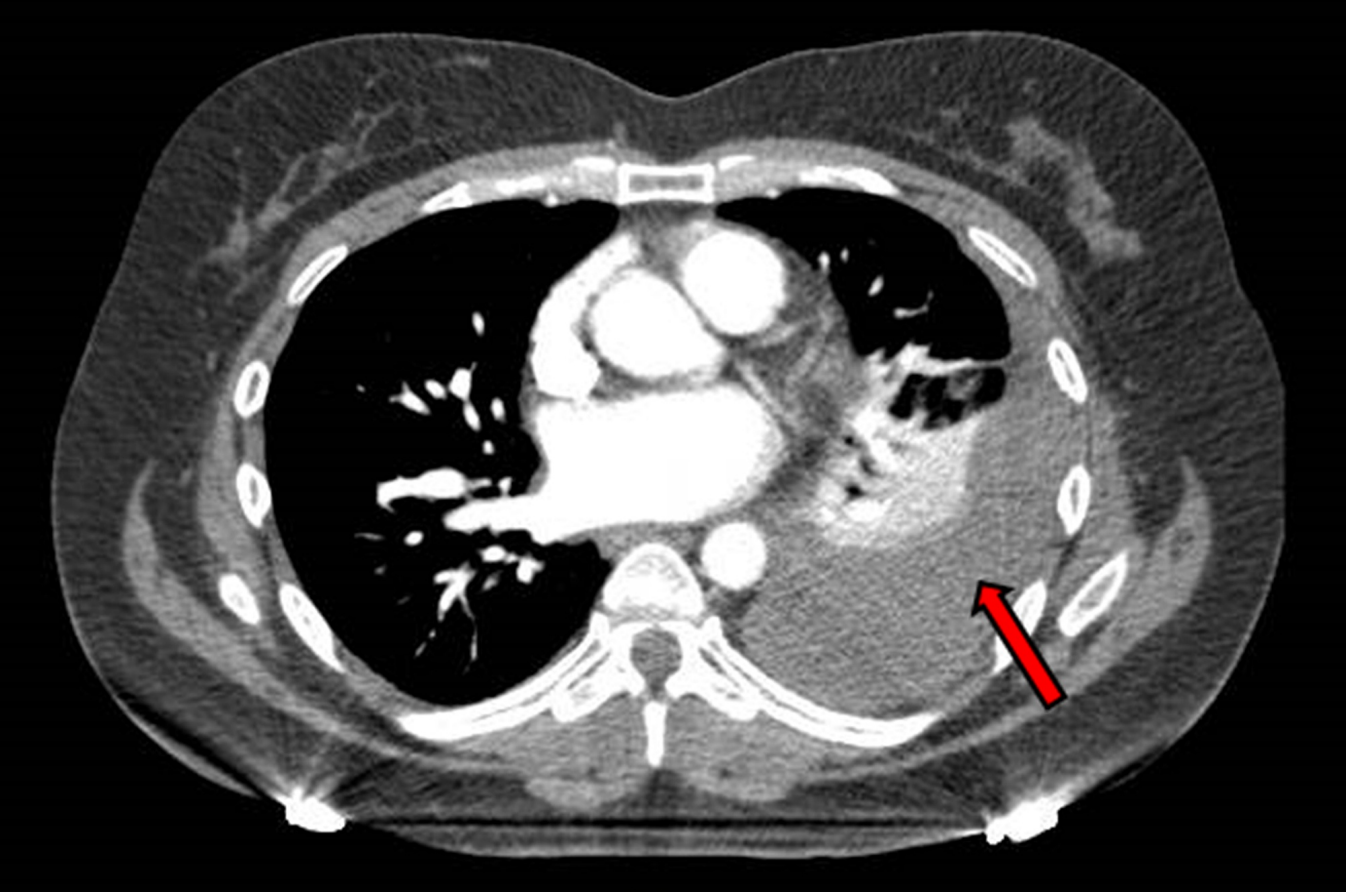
CT of the chest showing moderate to large left pleural effusion with left lung atelectasis (red arrow).
